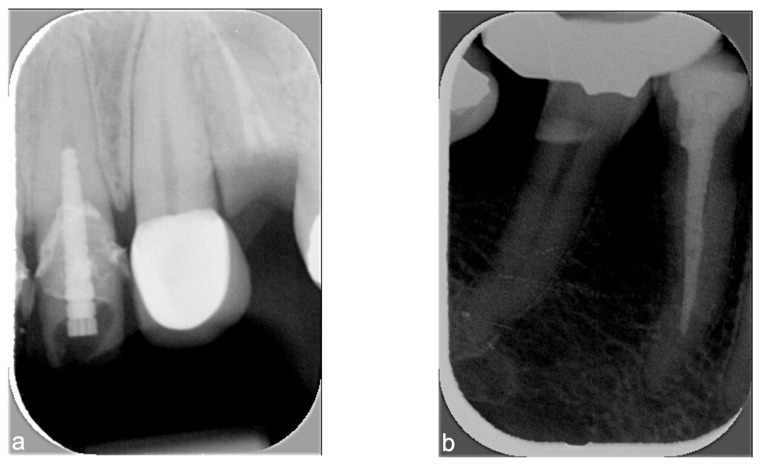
Periapical radiographs taken without rectangular collimation. From left to right: (a) incorrect bite on the film-holder (apex has been “cut off”) and (b) an overexposure error.
